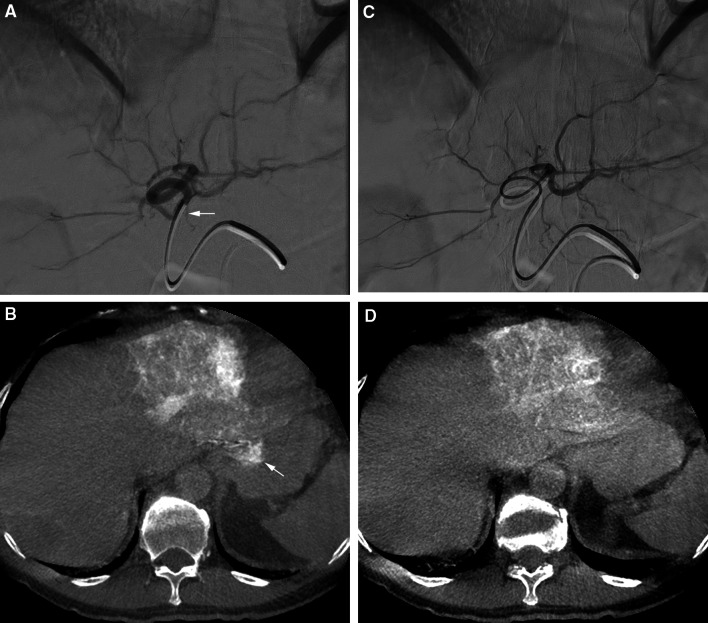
A DSA from the LHA. B C-arm CT performed from the LHA shows extrahepatic shunting in the gastric wall (*white arrow*). The small extrahepatic branch indicated by the *white arrow* in (A) was the culprit vessel. C The catheter was positioned more distal in the LHA. D C-arm CT performed from the new injection position did not show extrahepatic shunting anymore
